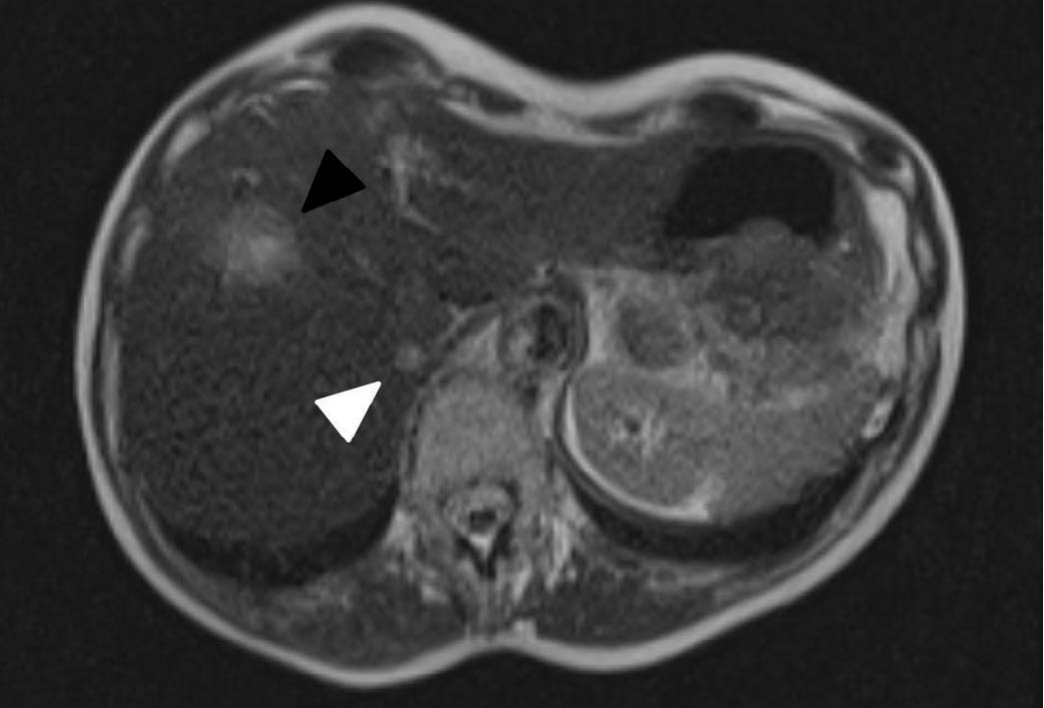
Axial T2 HASTE MRI showing 2.2-cm lesion in segment 8 (black arrow) and 0.8cm lesion in Segment 7 (white arrow).
